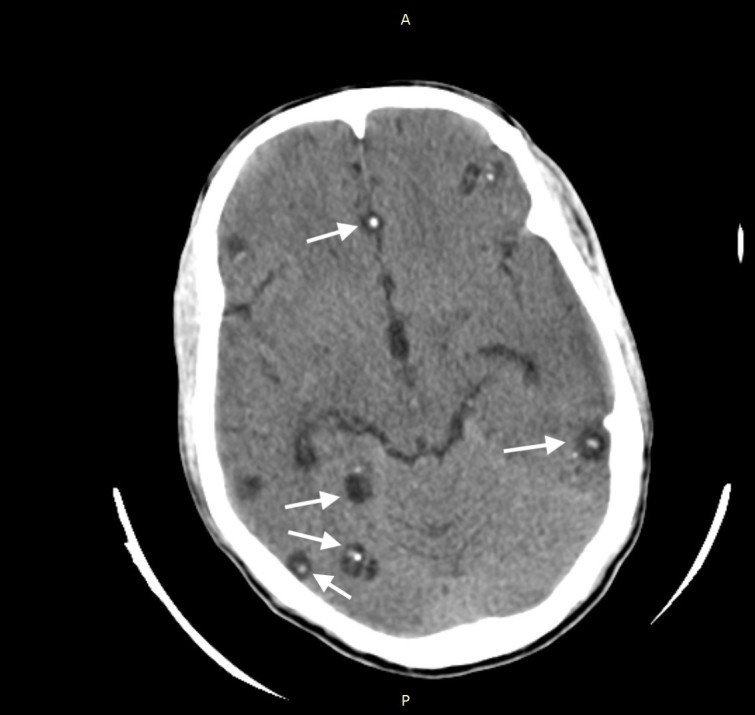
Computed tomography of the brain showing multiple cystic lesions and cyst with dot sign in patient with neurocystocercosis (arrows).
